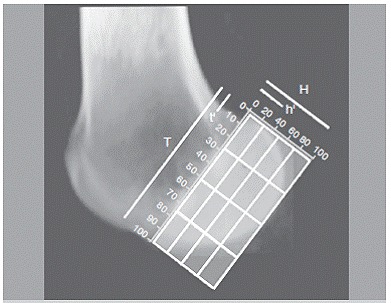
Bernard and Hertel^4^ quadrant method in a neutral transparent CT scan of the lateral femoral condyle. T = total condyle length, t'= central ACL percentage of T; H = total height, h' = central ACL percentage of H.
